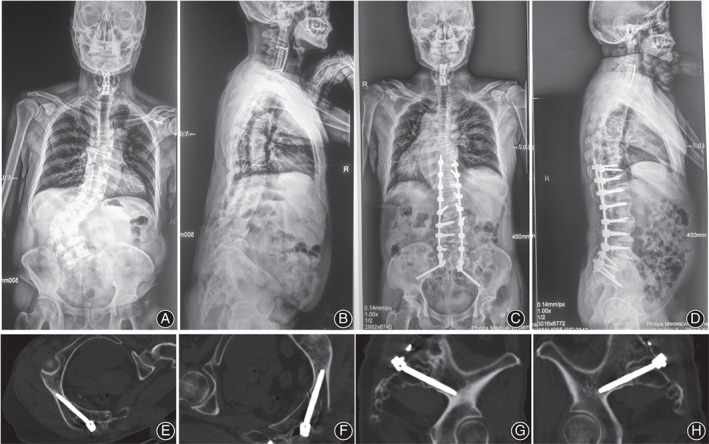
Typical case 3 managed by ball tip technique. A 68‐year‐old male presented severe back pain and weakness of low limbs. Rigid lumbar scoliosis. He underwent an anterior cervical discectomy and fixation (ACDF) at local hospital 5 years ago. Preoperative X‐rays showed a rigid lumbar scoliosis and asymmetric pelvis (A–D). Surgeon adopted the ball tip technique for S2AI screw placement. Postoperative X‐rays showed the satisfactory correction and good sagittal and coronal realignments with a sacropelvic fixation (Fig. 3C, D). Postoperative CT scan indicated the perfect positions of S2AI screws bilaterally in the axial and sagittal plates of screws without any cortex breach (E–H).
